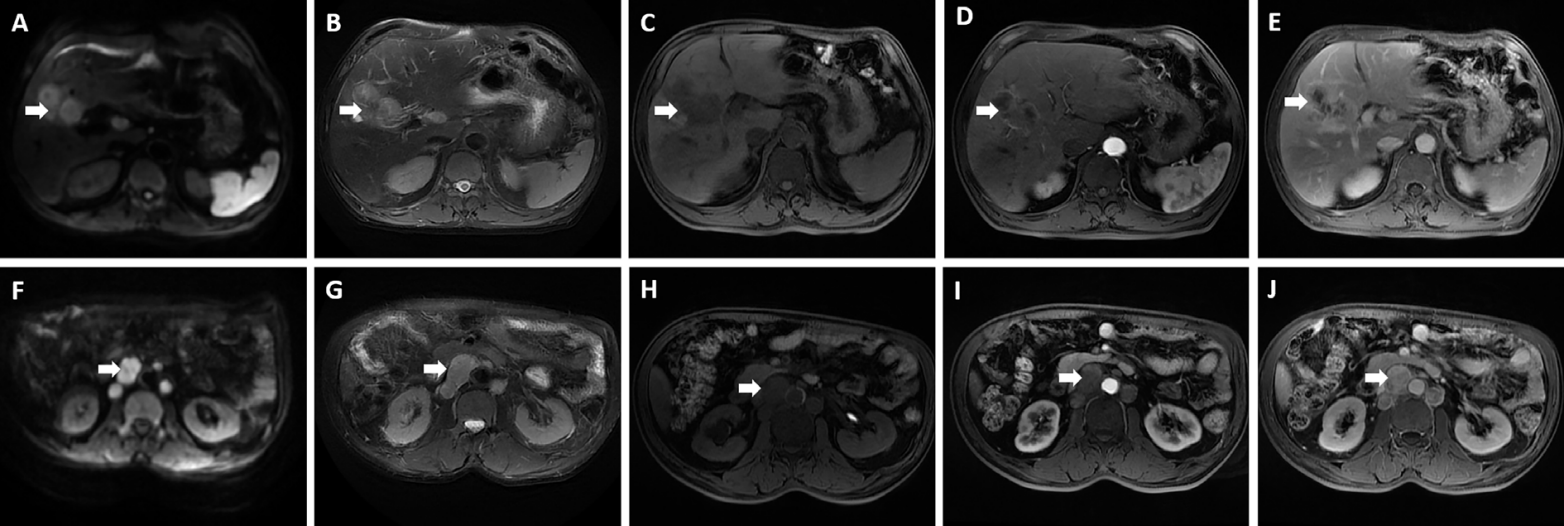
Magnetic resonance imaging showed an irregular morphology mass, with low signal in T1-weighted imaging, high signal in T2-weighted imaging, and limited diffusion in diffusion-weighted imaging (DWI); enlarged hepatic portal and retroperitoneal lymph nodes were also revealed—the larger one was 30.4 mm * 23.1 mm in size. (A, F) DWI; (B, G) T2-weighted imaging; (C, H) T1-weighted imaging; (D, I) The arterial phase; (E, J) The delayed phase. The white arrowheads direct the lesion or enlarged lymph nodes.
